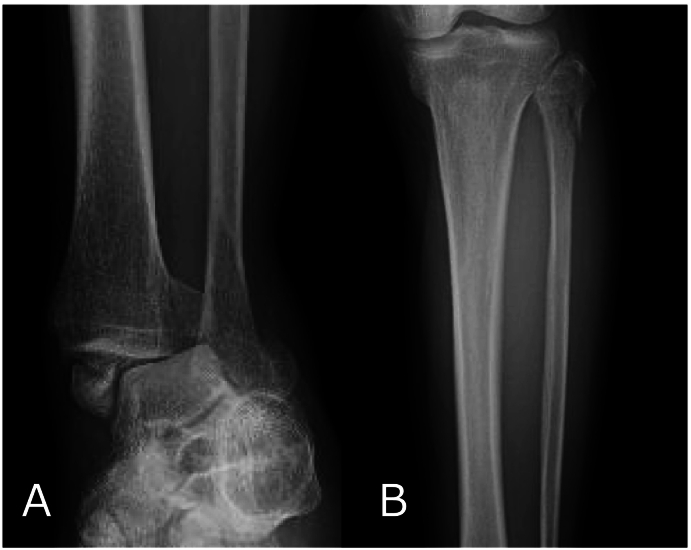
Preoperative X-ray findings. A) Anterior posterior view of the ankle. B) Anterior posterior view of the proximal lower leg.
